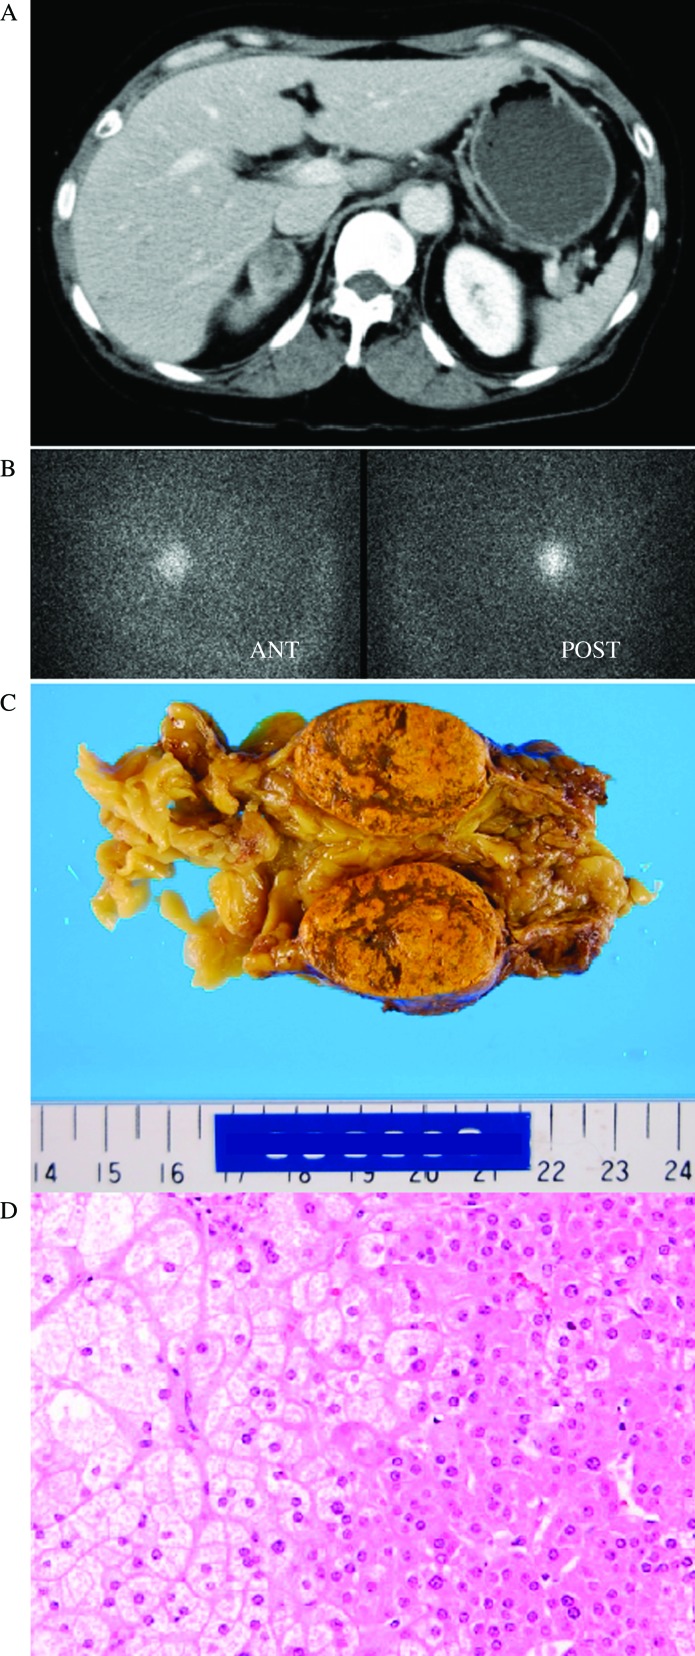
Abdominal computed tomography showing a 31×24 mm low-density mass within the right adrenal gland with mild enhancement post-contrast (A) that was also positive for [^131^I]adosterol imaging (B). Cut sections of the yellow adrenocortical adenoma (30×30×20 mm, 25 g nodule) with the atrophic right adrenal gland (C). Pathological evaluation of the right adrenal mass indicated diagnostic characteristics of a typical adrenocortical adenoma with Cushing's syndrome (D).
